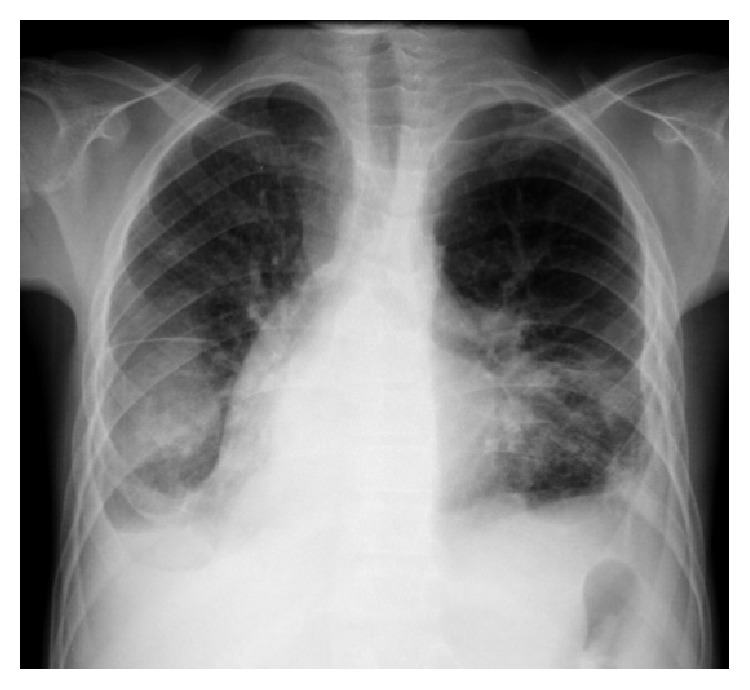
Posteroanterior chest radiography: mild cardiac dilation and bilateral pulmonary effusion, predominantly in the right hemithorax.
